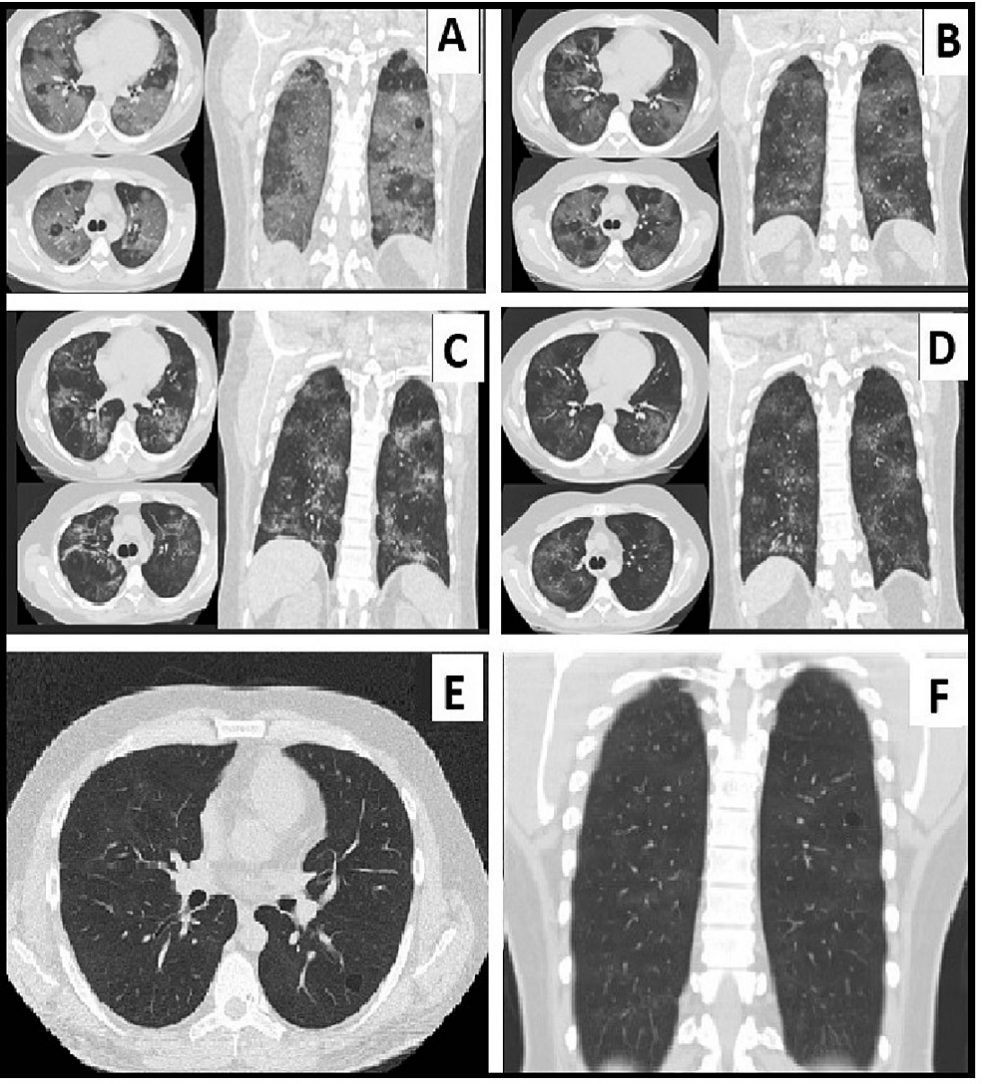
Dynamics of the radiological changes on chest CT A - axial and coronal views with widespread ground-glass opacities at presentation, B - axial and coronal views on day 5 after the initiation of therapy, C - axial and coronal views on day 11 after the treatment, D - axial and coronal views on patient’s discharge (day 27), E - axial view and F - coronal view - outpatient follow-up images showing a complete resolution of infiltrative changes
